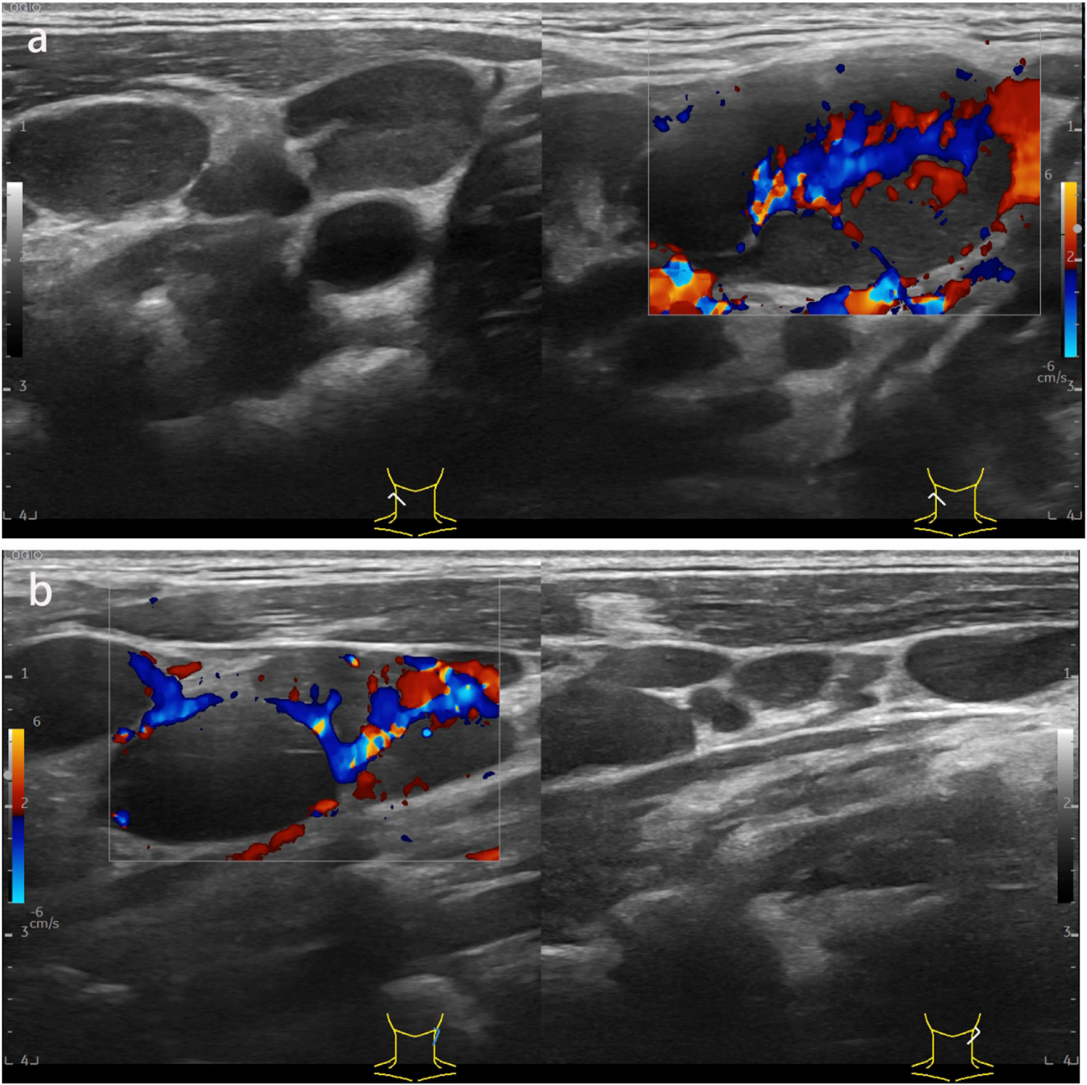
(a and b) Grey scale ultrasound images of the neck demonstrating multiple enlarged hypoechoic lymph nodes bilaterally with areas of partial liquefaction and necrosis.
